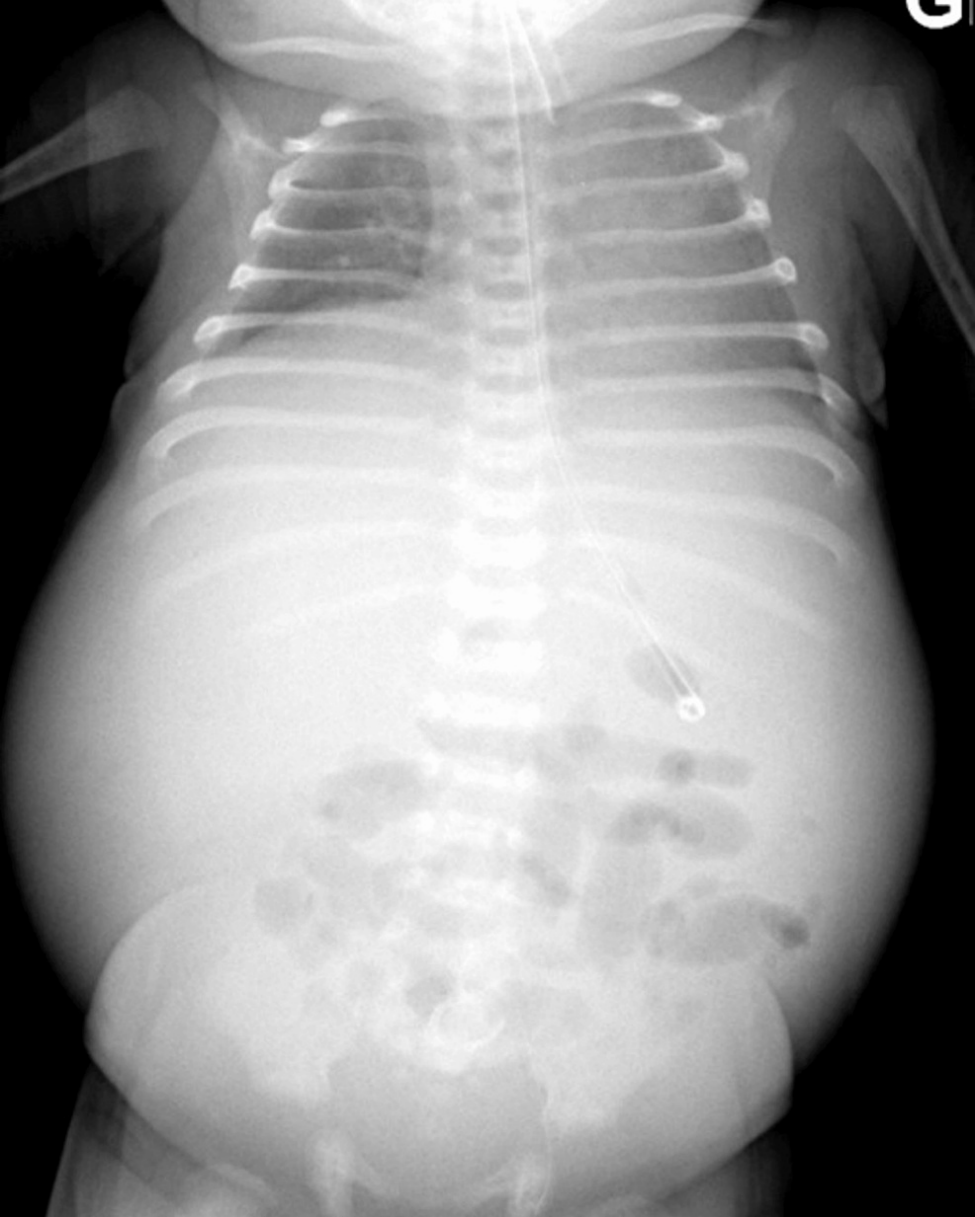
Supine abdominal x-ray at day 11
